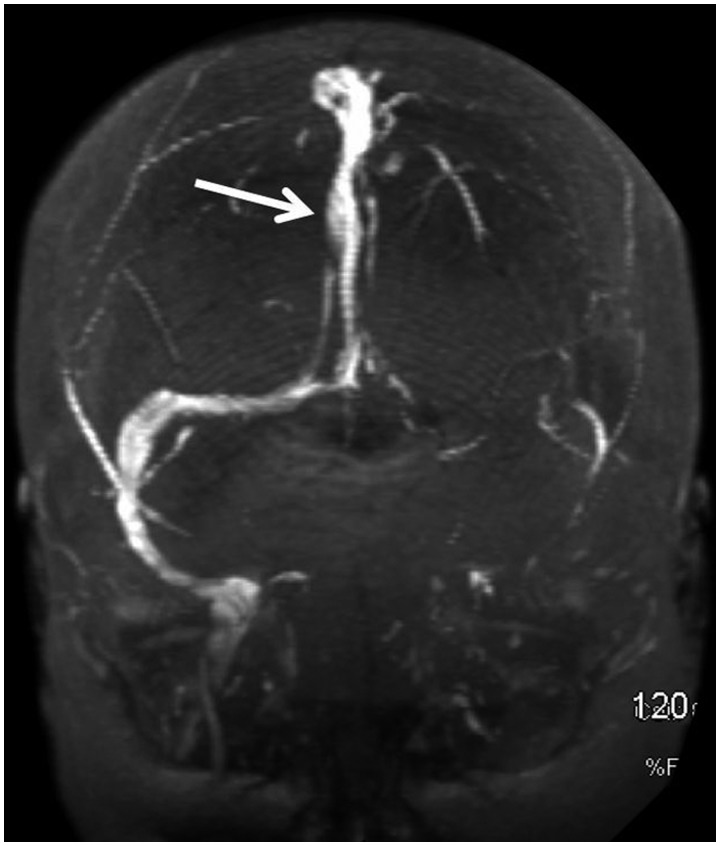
Magnetic resonance imaging with venography prior to discharge, demonstrating marked recanalization and improved flow signal (arrow).
